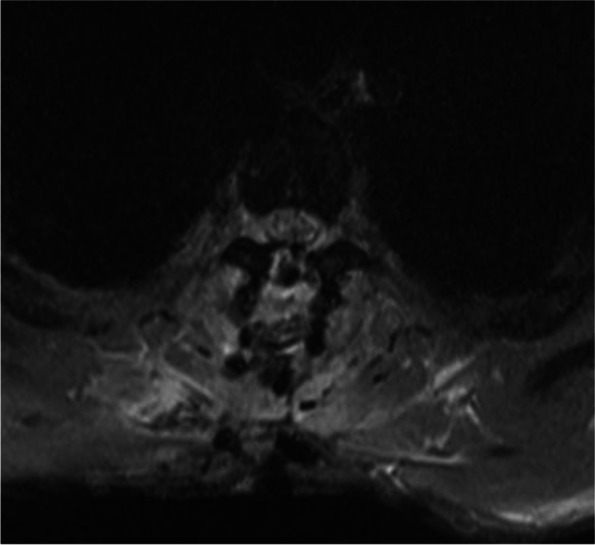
Immediate Post-Operative MRI Axial T2 weighted MRI at T2/3 level demonstrating the cord high signal in the repaired area
